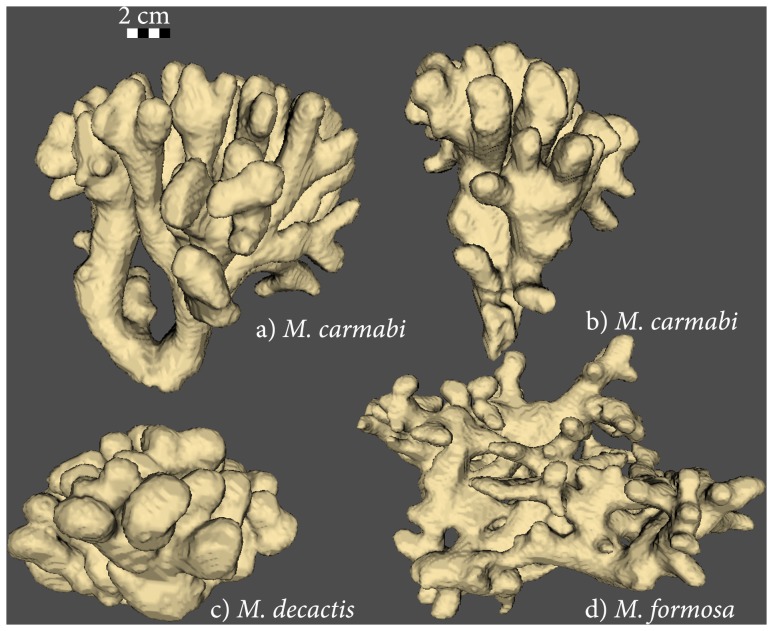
CT-scans of four Madracis colonies. Volume rendering of the CT-scans of the Madracis coral colonies: a) *M. carmabi*, b) *M. carmabi* sample Car436 in the data set, c) *M. decactis* and d) *M. formosa.*
